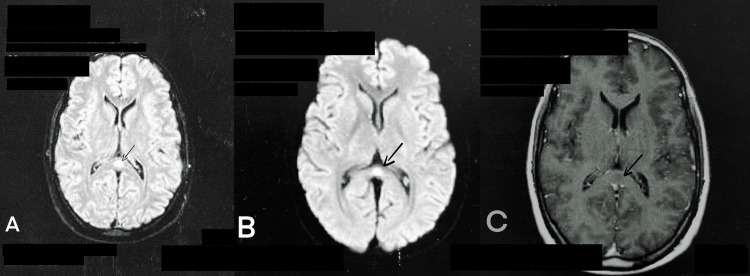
Brain MRI obtained on admission (axial view) (A) A hyperintense lesion in the splenium of the corpus callosum (SCC) on fluid‐attenuated inversion recovery images (FLAIR) (black arrow); (B) A hyperintense lesion in the SCC on diffusion-weighted imaging (DWI) (arrow); (C): no contrast enhancement on T-1 weighted images (arrow)
